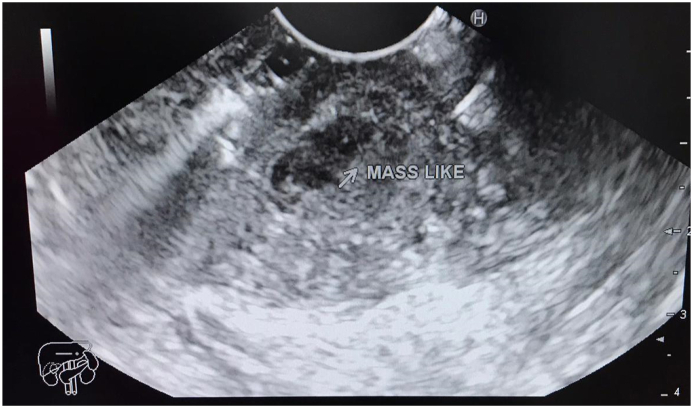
shows Mass-like lesion found on Endoscopic Ultrasound.
